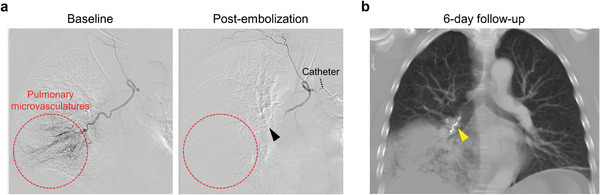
Limited access of Obsidio™ conformable gel embolic to the distal microvessels. a) Digital subtraction angiography (DSA) in a 63‐year‐old male with acute and severe hemoptysis secondary to lung adenocarcinoma; red circle indicates the area of hemorrhage. The patient underwent bronchial arterial embolization using Obsidio gel embolic, resulting in instant hemostasis (black arrow; red circle). The gel embolic successfully embolized the upstream larger bronchial artery and did not address the smaller bleeding blood vessels distally. b) The computed tomography (CT) chest angiogram at 6‐day follow‐up revealed a stable Obsidio gel embolic (yellow arrow) but confirmed that the peripheral microvasculatures were not directly targeted. A few days after embolization, bleeding resumed from the distal microvessels eventually requiring an elective surgical resection.
